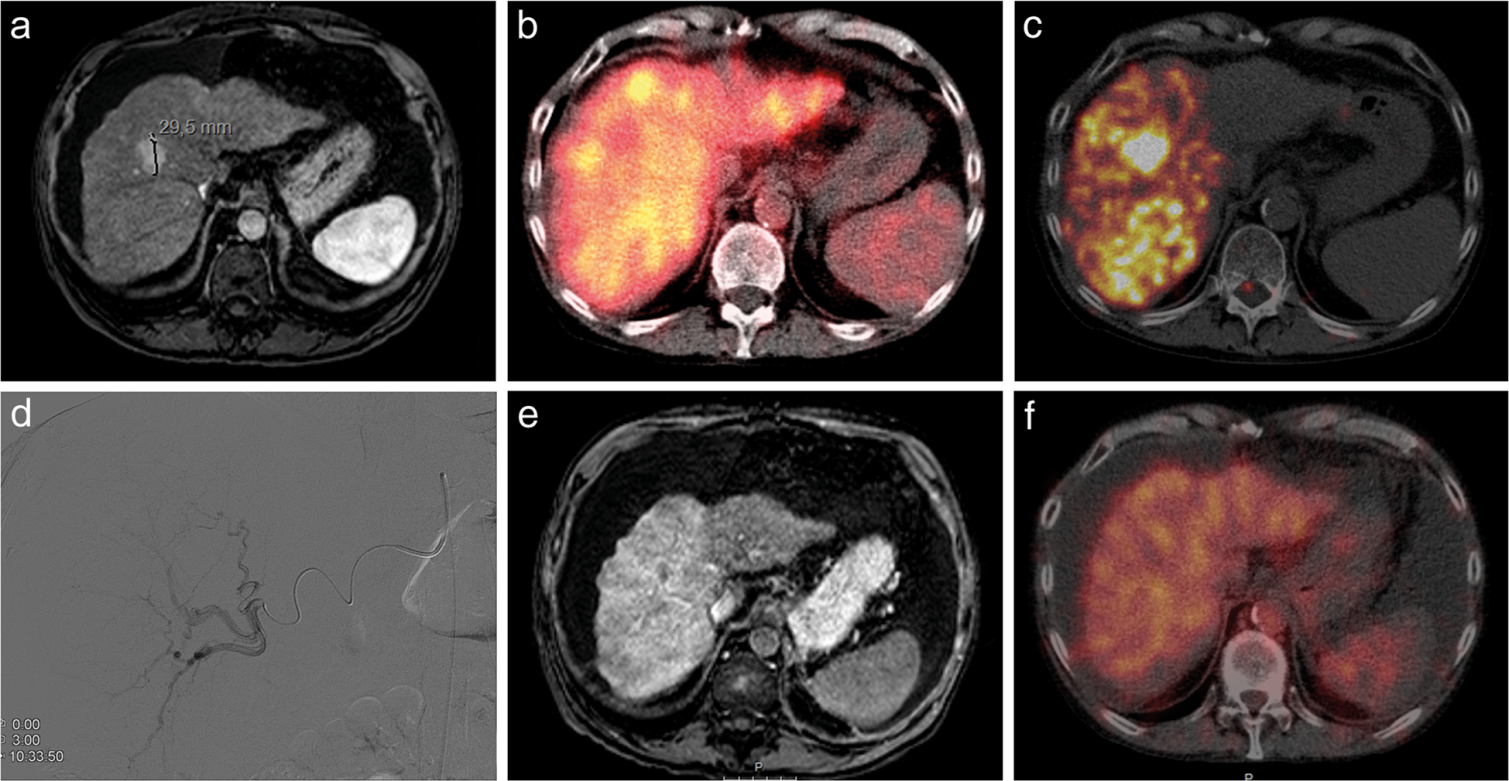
Pre- and posttreatment images of case 2. On the arterial phase of the pretreatment MRI, a hypervascular lesion in segment 8 is depicted on a background of liver cirrhosis, consistent with a HCC (a). Pretreatment hepatobiliary scintigraphy shows a visually fairly homogenous ^99m^Tc-mebrofenin uptake with a defect in segment 8, corresponding to the HCC (b). Posttreatment ^90^Y-PET/CT shows good targeting of the HCC (227 Gy), but also a significant ^90^Y deposition in segment 7 (91 Gy) (c). At treatment angiography, the endhole catheter tip was positioned at the bifurcation of the anterior and posterior right hepatic artery. The overdosage of the posterior sector is therefore most likely due to preferential flow (d). Posttreatment MRI and hepatobiliary scintigraphy show ascites and shrinkage of the cirrhotic liver with increased arterial enhancement of the treated lobe and decreased ^99m^Tc-mebrofenin uptake (e, f)
